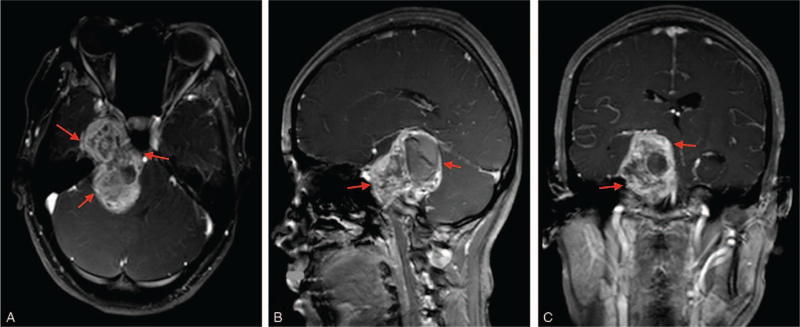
(A & B): Are preoperative MRI showing an extra-axial mass with mixed signal intensities in the right petroclivus. A = axial view, B = sagittal view, C = coronal view*. Red arrow = tumor*.
